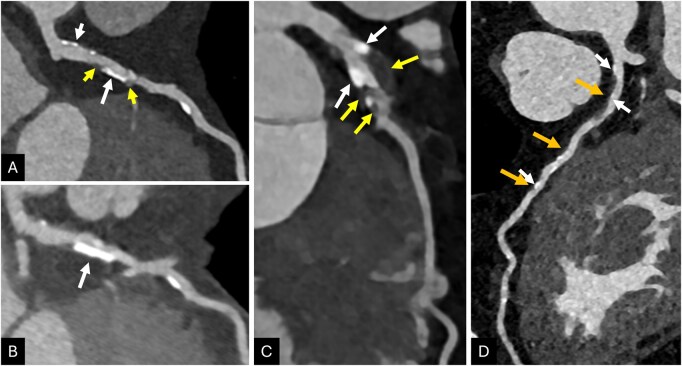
An 82-year-old male underwent 2 coronary CT angiography examinations obtained 2 months apart (A, PCD-CTA; B, EID-CTA), demonstrating a left anterior descending (LAD) artery with multifocal calcified plaque in the proximal and mid segments. Note that on EID-CT (B), focally moderate stenosis is shown adjacent to a calcific plaque (white arrow); however, PCD-CT (A) in the same region shows only mild stenosis (white arrow) and improved visualization of noncalcified (yellow arrow) components. A 77-year-old female underwent PCD-CT coronary angiography (C), showing a large complex plaque including calcified (white arrows) and noncalcified (yellow arrows) components in the proximal LAD with moderate luminal stenosis. A 68-year-old male underwent PCD-CT coronary angiography (D), showing a predominantly soft plaque in the proximal and mid LAD (orange arrows) with spotty calcifications (white arrows). Abbreviations: CTA, CT angiography; EID, energy-integrating detector; PCD, photon-counting detector.
